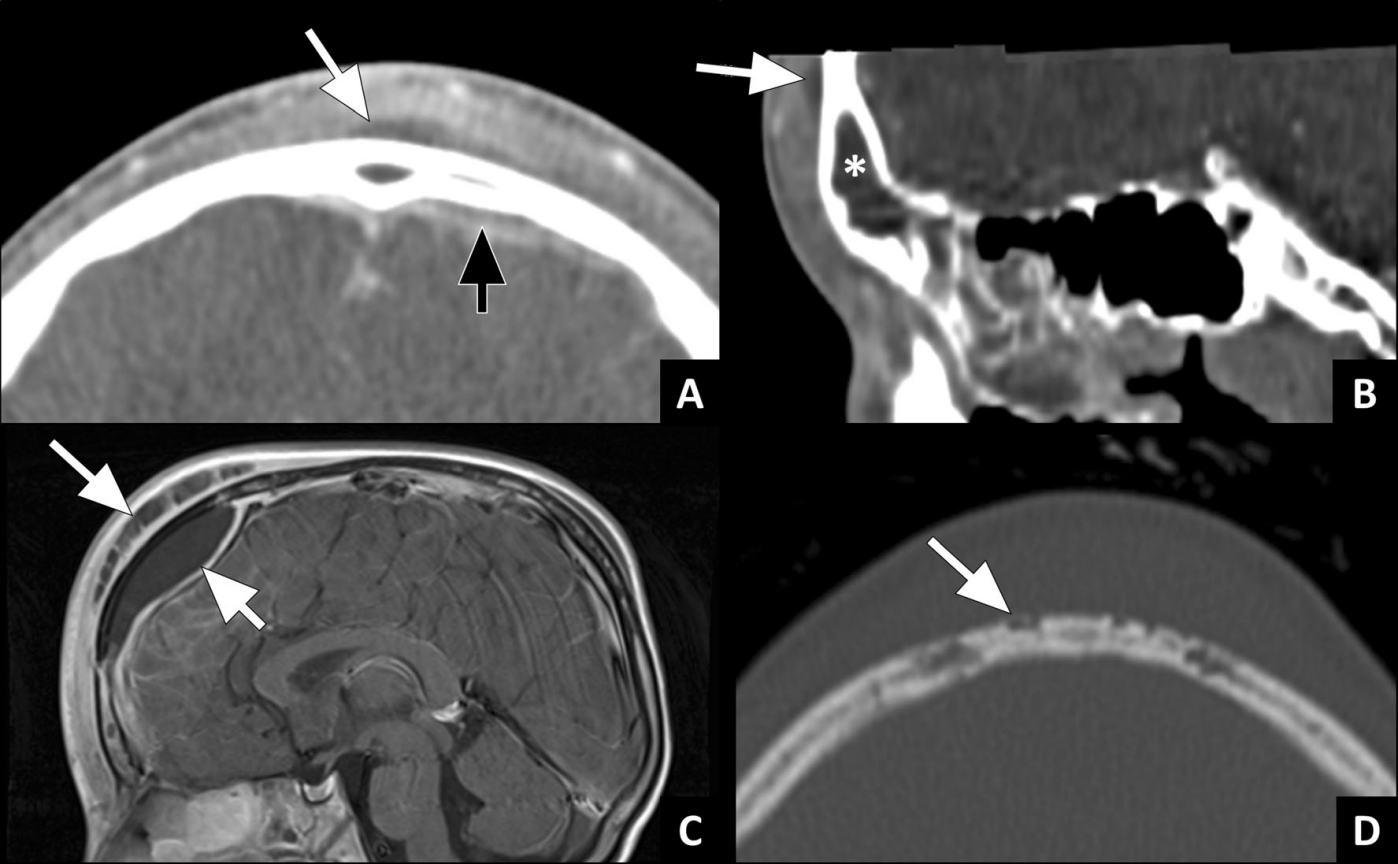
Pott’s puffy tumour: a) Axial and b) sagittal ceCT image in a soft tissue window showing opacification of the frontal sinus with heterogeneous material (*) and a subperiosteal abscess (white arrow) due to frontal sinusitis. Note the small subdural fluid collection (black arrow) indicating subdural empyema. c) Sagittal T1w ceMRI in another patient showing a large subperiosteal abscess (long arrow), and a large extra-axial empyema (short arrow). d) Axial CT image in a bone window in the same patient showing erosion of the tabula externa (arrow) adjacent to the soft tissue mass, indicative of osteomyelitis of the frontal bone
